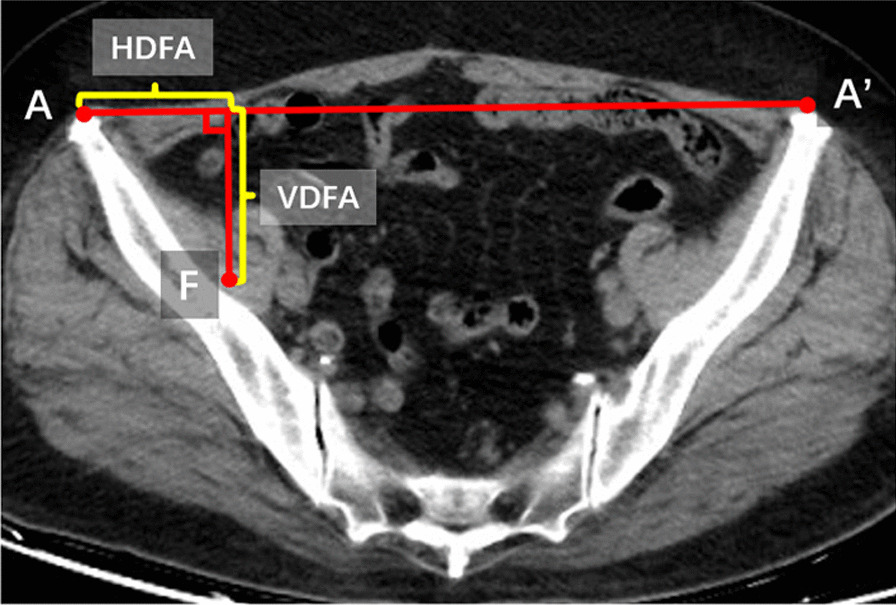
Relevant parameters were measured on CT radiograph before operation with the extramedullary femoral cutting system. A/A′: Anterior superior iliac spine. F: The femoral head center
